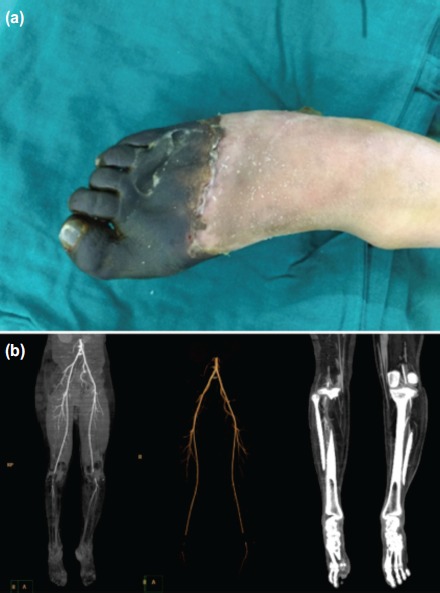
(a) Clinical picture of right foot showing gangrene of right forefoot and (b) CT angiogram of both lower limbs showing thrombosis of popliteal arteries.
